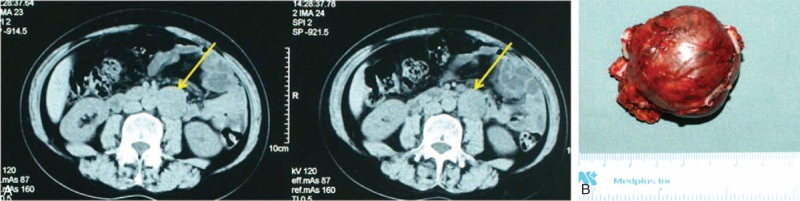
A. Computed tomography (CT) revealed retroperitoneal paraganglioma in the left side. B. General photograph of paraganglioma.
